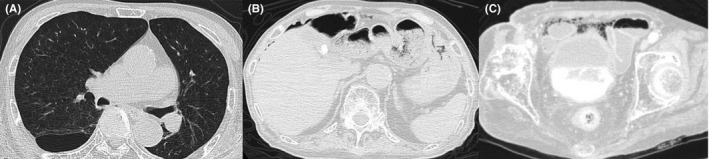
Radiological examination of Case 3. A, Computed tomography showed remarkable pulmonary emphysema. B, C, Computed tomography showed intra‐abdominal free air around the liver surface and in the pelvic cavity
